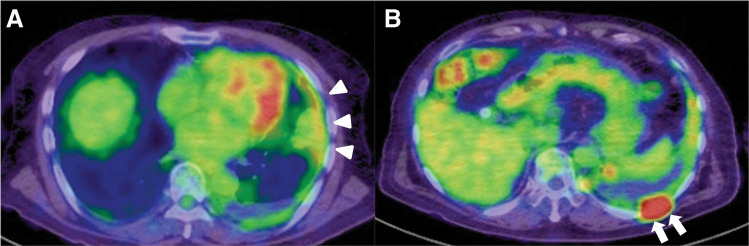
Positron emission tomography/computed tomography (PET-CT) performed 10 months prior to the emergent cardiac surgery. (A) Nodular-to-cordiform accumulation over a wide area of the left pleura (arrowheads). No obvious intra-atrial accumulation was observed. (B) Mass-like accumulation in the dorsal aspect of the left tenth intercostal space (arrows).
